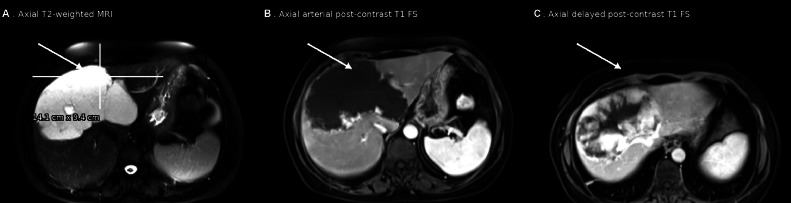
Baseline MRI examination (February 2022). (A) Axial T2-weighted image demonstrates a markedly hyperintense giant hepatic hemangioma; white arrows indicate the lesion and measurement lines show transverse and anteroposterior dimensions of 14.1 × 9.4 cm. (B) Axial arterial-phase post-contrast T1-weighted fat-suppressed image demonstrates peripheral nodular discontinuous enhancement. (C) Axial delayed postcontrast T1-weighted image demonstrates progressive centripetal fill-in characteristic of hepatic hemangioma.
